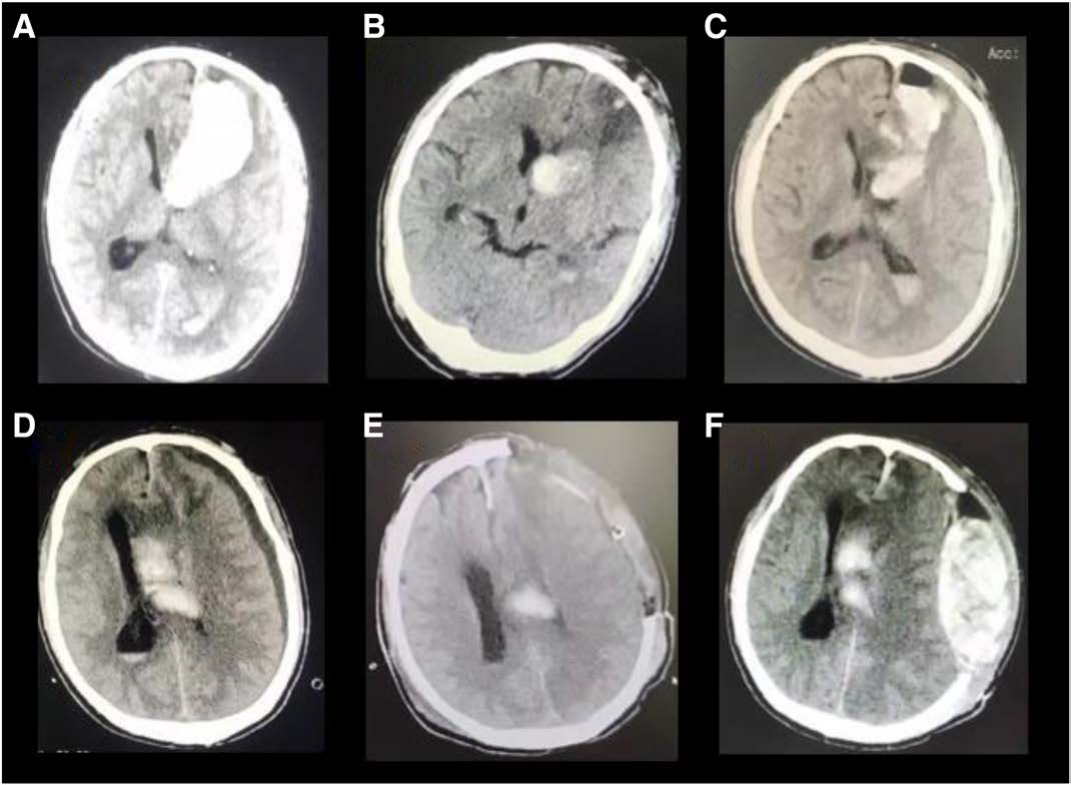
Cranial CT imaging from the referring hospital. (A) Day 1: Left frontotemporal lobe hemorrhage on admission. This explains the patient dizziness and headache (B) Post-first surgery follow-up scan: Reduced hematoma volume in the left frontotemporal region. The patient consciousness has improved. (C) Postoperative day 4 follow-up scan: New-onset hemorrhage in the left frontotemporal lobe. The patient consciousness is blurred again. (D) Postoperative day 7 follow-up scan: Left lateral ventricular hemorrhage with left subdural effusion. (E) Post-second surgery follow-up scan: Decreased intraventricular hemorrhage volume compared to prior imaging. (F) Post-second surgery day 4 follow-up scan: Left frontotemporal epidural and subdural hematomas causing midline shift due to mass effect from the hematoma. The patient is unconscious. CT = computed tomography.
